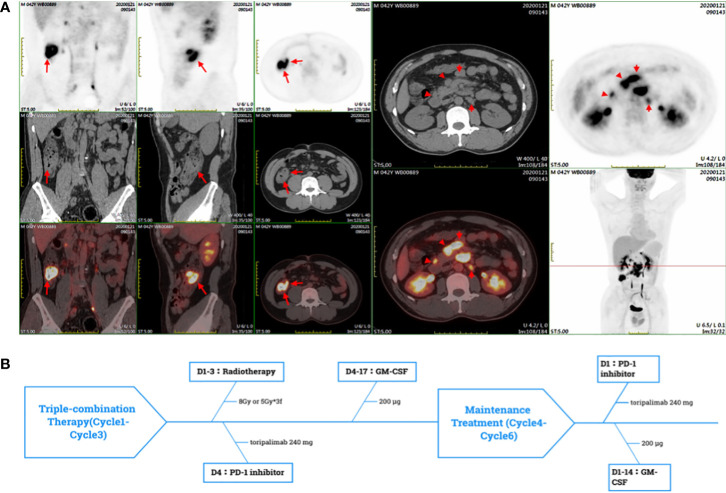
(A) The PET-CT showed the high SUV_mean_ of ascending colon and enlarged lymph nodes of retroperitoneal and peritoneal. (B) In a PRaG cycle, radiotherapy was delivered for metastases, followed by GM-CSF subcutaneous (sc) injection once daily for two weeks, and toripalimab was intravenous(iv) once within one week after radiotherapy. PRaG Therapy was repeated every three weeks, and three cycles of triple-therapy were administered. Subsequently, the patient underwent three cycles of PD-1 inhibotor and GM-CSF maintenance treatment.
